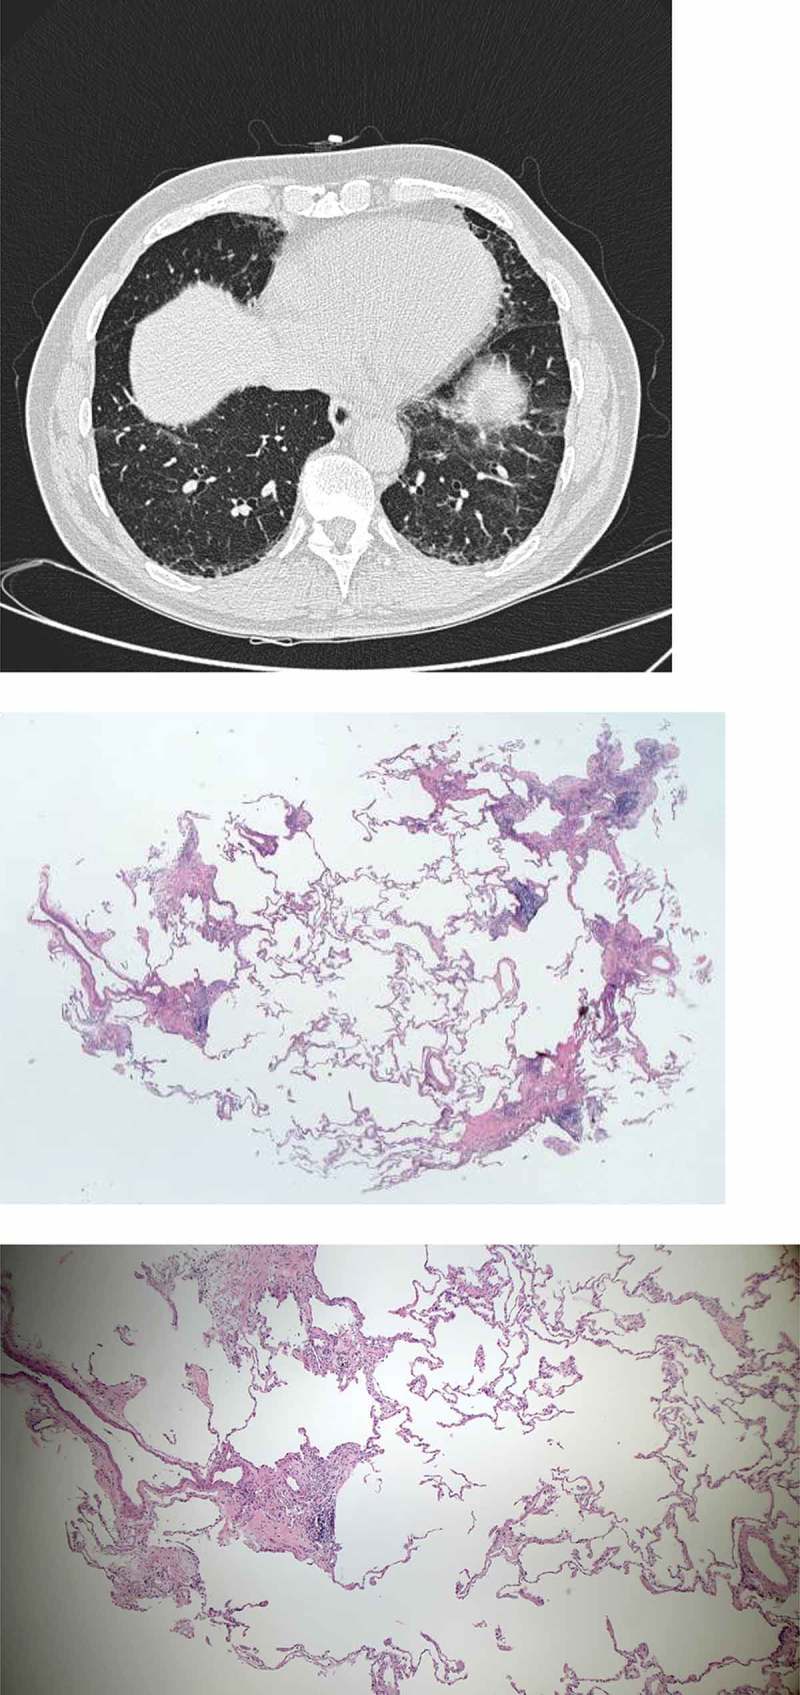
71-year-old male referred for dyspnea. HRCT showing reticulation, ground glass opacity and traction bronchiectasies with basal predominance. Cryobiopsies showing patchy fibrosis, fibroblastic foci and chronic inflammation. The patient was diagnosed with idiopathic pulmonary fibrosis, high confidence.
